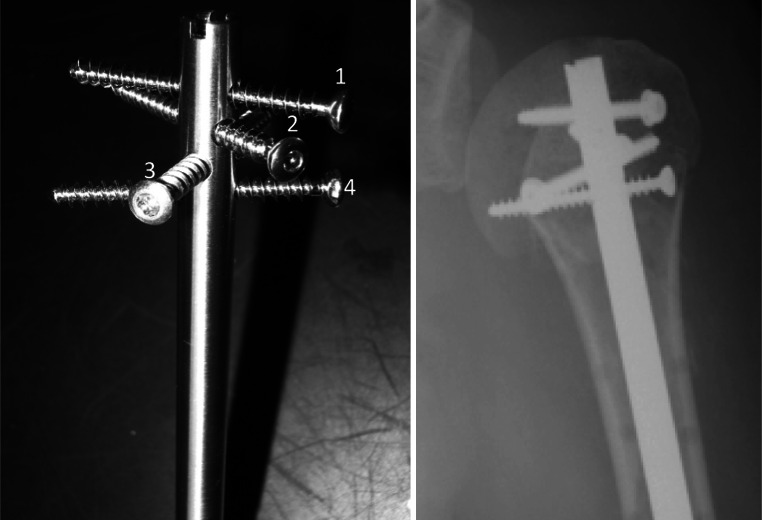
T4 nail, proximal end and X-ray
